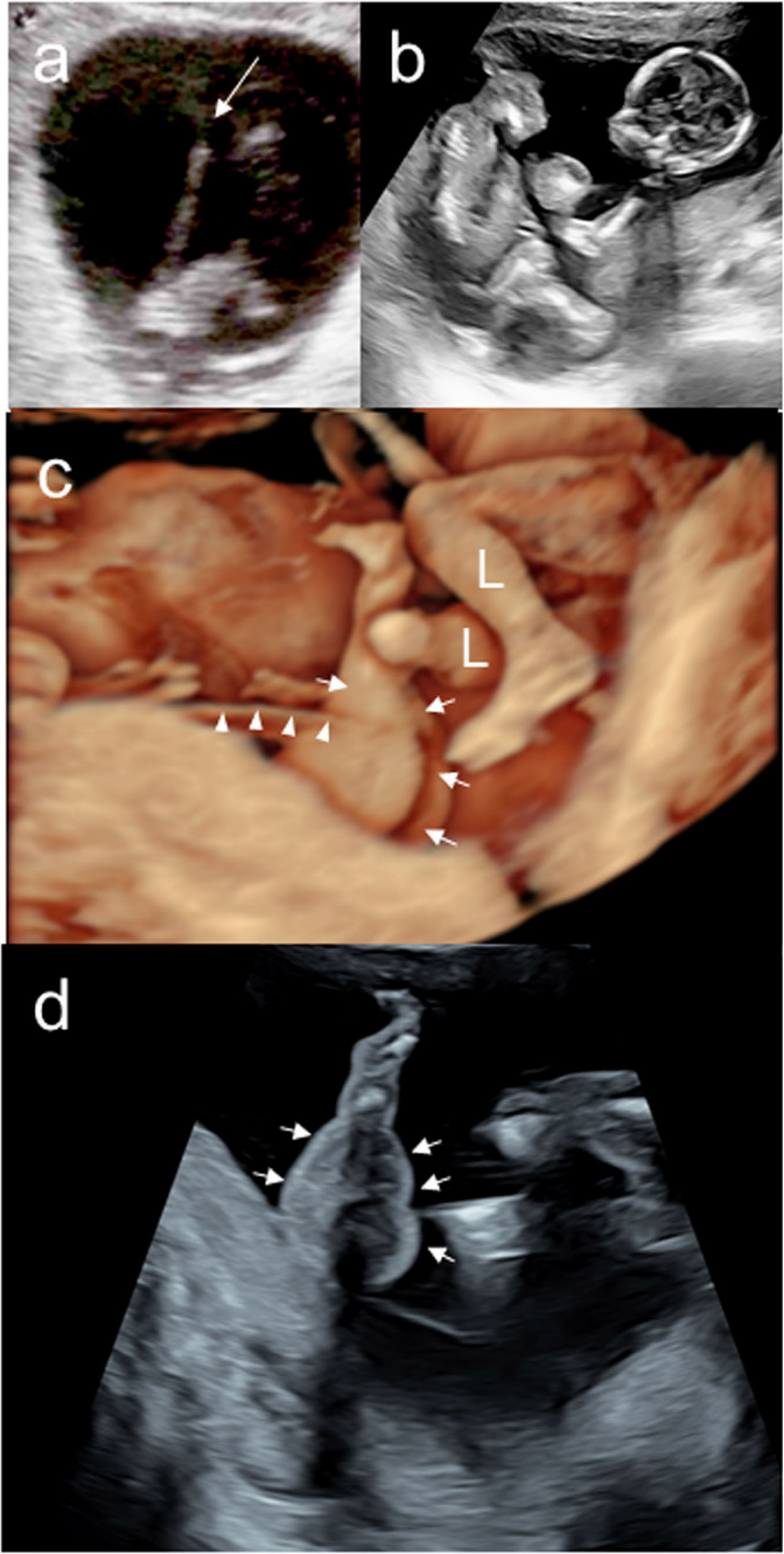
Fetal ultrasonography. a Transvaginal ultrasonography at 8 weeks of gestation. The lack of the “twin peak” sign, also called the lambda sign (arrow), indicates an MCDA twin pregnancy. b Transabdominal fetal ultrasonography at 15 weeks of gestation. There is no evidence of a dividing membrane. c Transabdominal three-dimensional ultrasonography at 18 weeks of gestation. Baby B has a swollen upper left arm (arrows), and part of the amniotic membrane (arrowheads) is attached to this arm. *L* legs of baby A, *P* placenta. d Transabdominal two-dimensional ultrasonography at 18 weeks of gestation. Baby B has a swollen upper left arm (arrows)
